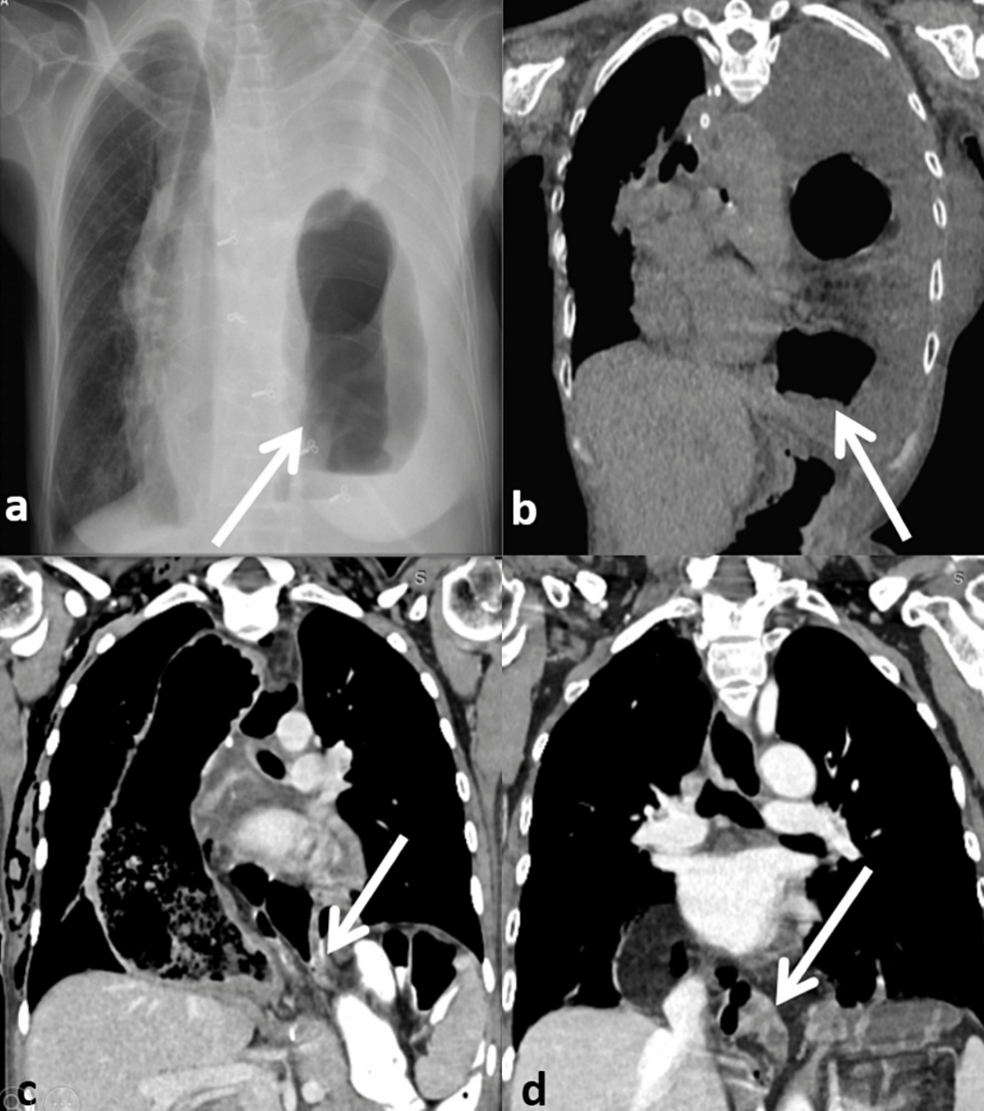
a and b - Chest X-ray and coronal view of HRCT chest of Case 1 respectively showing herniated dilated transverse colon loops with a compressed left lung and mediastinum shifted to the right. c and d - Coronal view of CECT of thorax and abdomen of Case 2 and Case 3 respectively showing herniated transverse colon loops. Solid white arrow shows herniated segment. HRCT, high resolution CT; CECT, contrast enhanced CT
